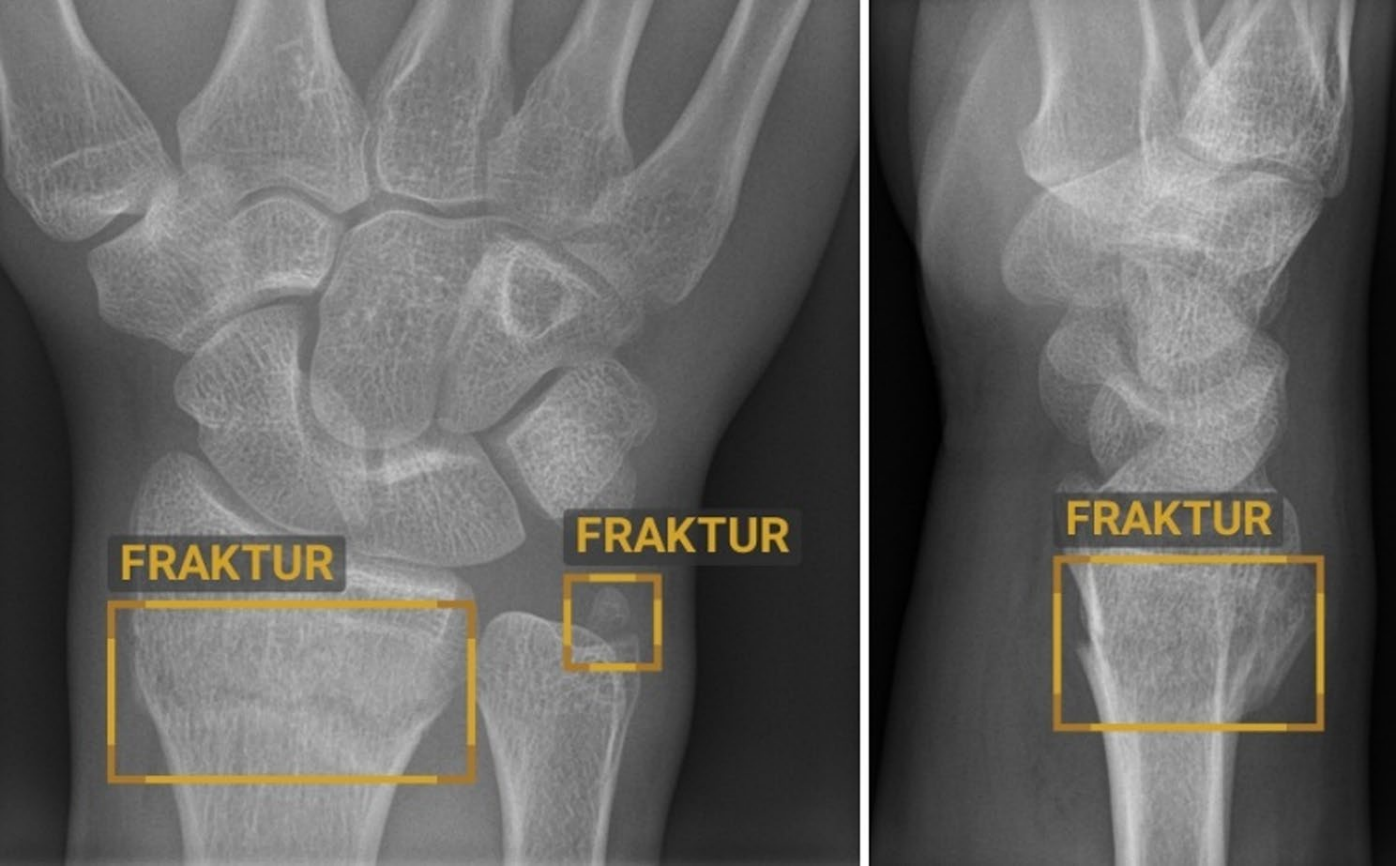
Example of AI-guided fracture detection on wrist radiographs. *AI: artificial intelligence*
